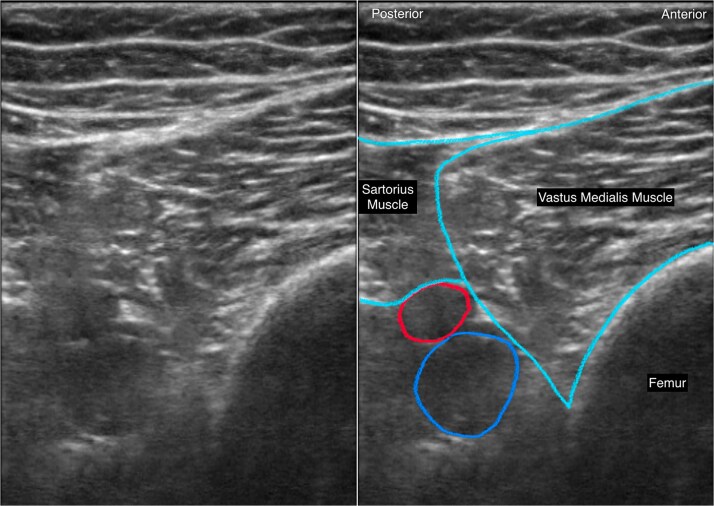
Ultrasound image of the anatomical region targeted in the proximal approach to infiltration between the popliteal artery and the capsule of the knee (p-iPACK) block. The ultrasound probe is placed transversely over the anteromedial thigh, ~1 fingerbreadth proximal to the base of the patella, and the block needle is inserted in the medial-to-lateral direction of which LA is injected into the interspace between the femur and the popliteal fossa. The femoral artery (red) and femoral vein (blue) are highlighted, with muscle borders outlined in cyan. Relevant muscles and bony structures are labeled.
